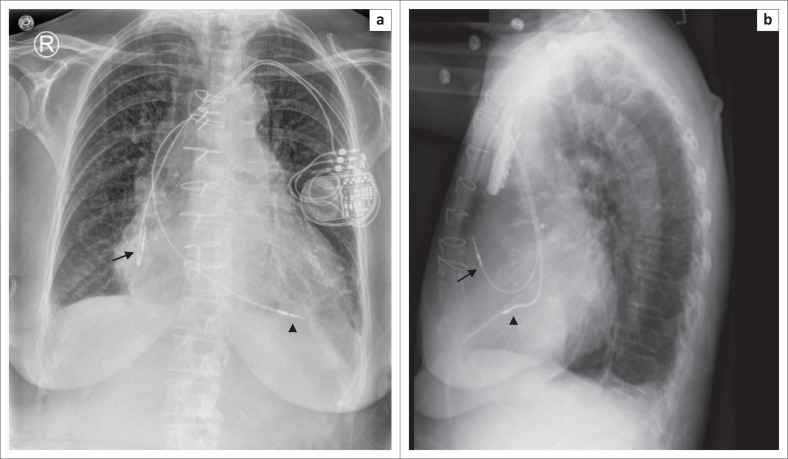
Frontal (a) and lateral (b) view chest radiographs showing a dual-chamber pacemaker with the right atrial lead (arrow) in the right atrial appendage forming a ‘J’ on the lateral view and the right ventricular lead (arrow head) pointing towards the cardiac apex.
